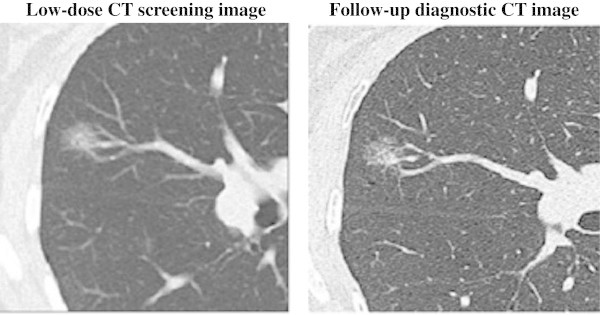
Low-dose CT screening image and follow-up diagnostic CT image. Low-dose CT screening image with a slice thickness and interval of 3 mm. Follow-up diagnostic CT image with an image slice thickness and interval of 1 mm. A 60-year-old woman with bronchioalveolar carcinoma (adenocarcinoma in situ) with mixed ground-glass opacity or pure ground-glass opacity in the right middle lobe (T1N0M0).
